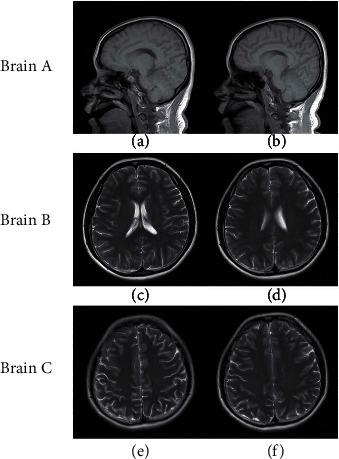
MR images used in the experiments: Brain A: the reference image (a) and target image (b); Brain B: the reference image (c) and target image (d); Brain C: the reference image (e) and target image (f).
